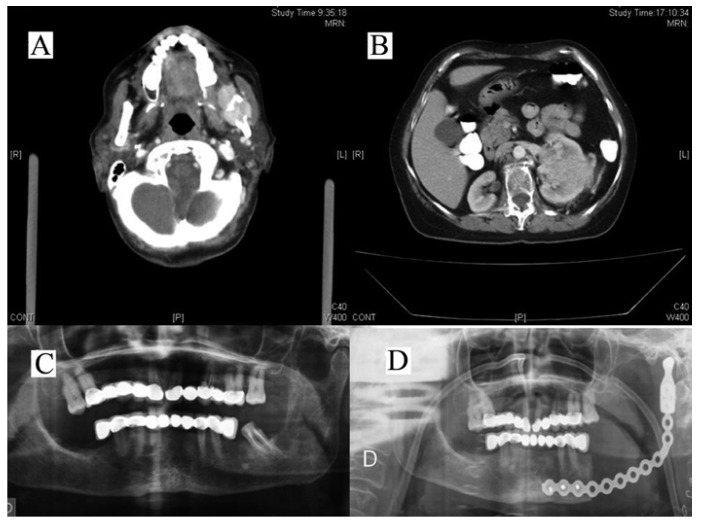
A, B) Cervical and abdominal CT showing synchronic metastasis of a hypernephroma in the left mandibular branch and primary tumour in the left kidney. C, D) Orthopantomography: metastatic radiolucent area in the left mandibular branch and plate reconstruction after hemimandibulectomy.
